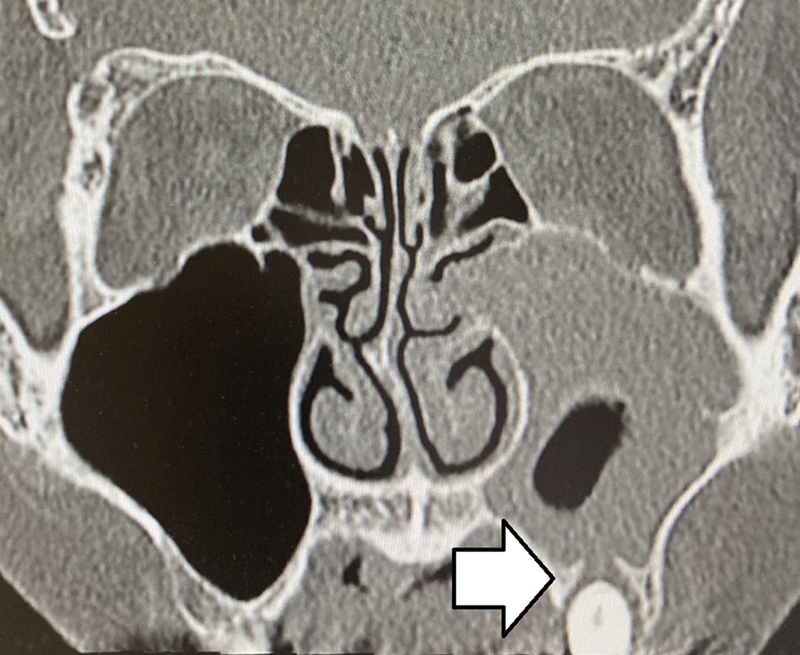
Computed tomography image of odontogenic sinusitis. Unilateral sinusitis with a root apex lesion is observed (white arrow).
